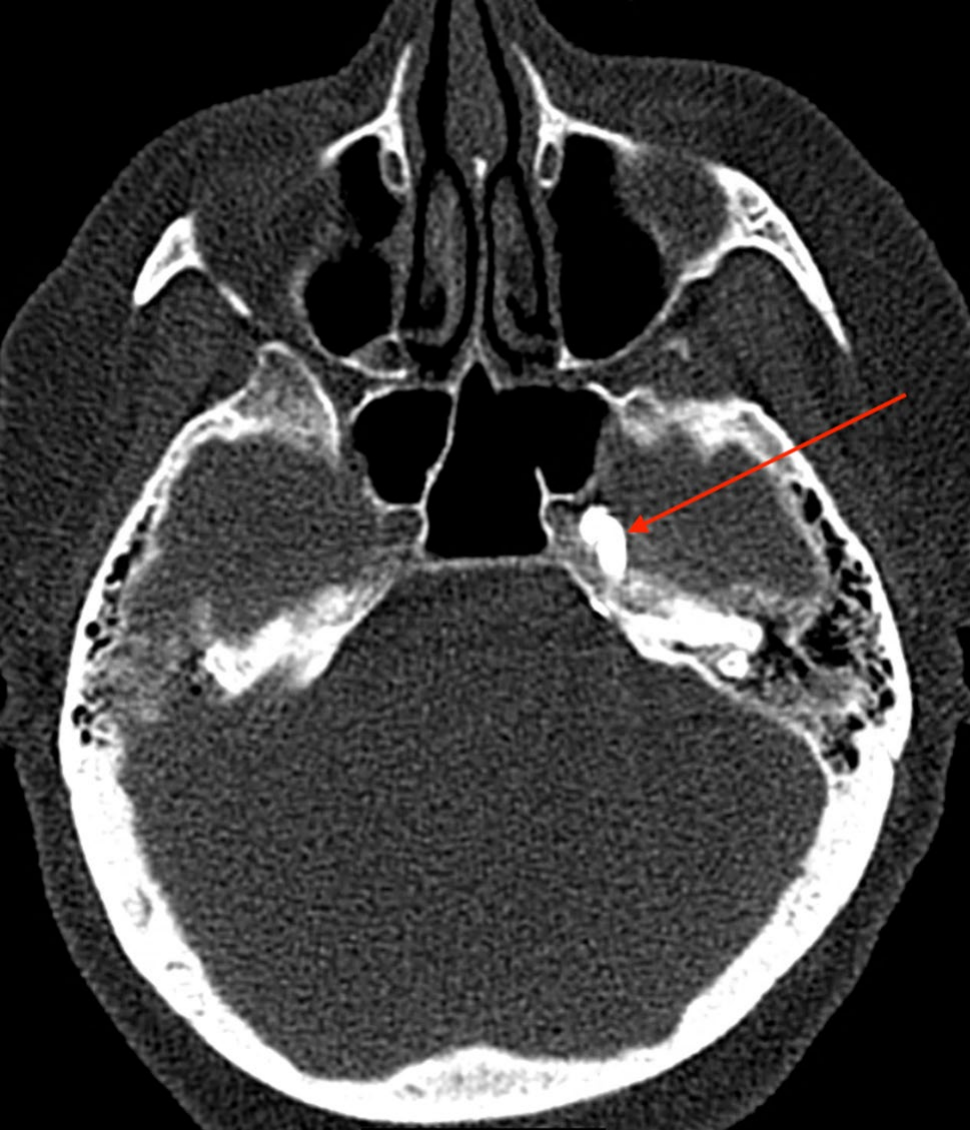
Red arrow showing contrast agent filling left-sided Meckel’s cave on axial CT scan
